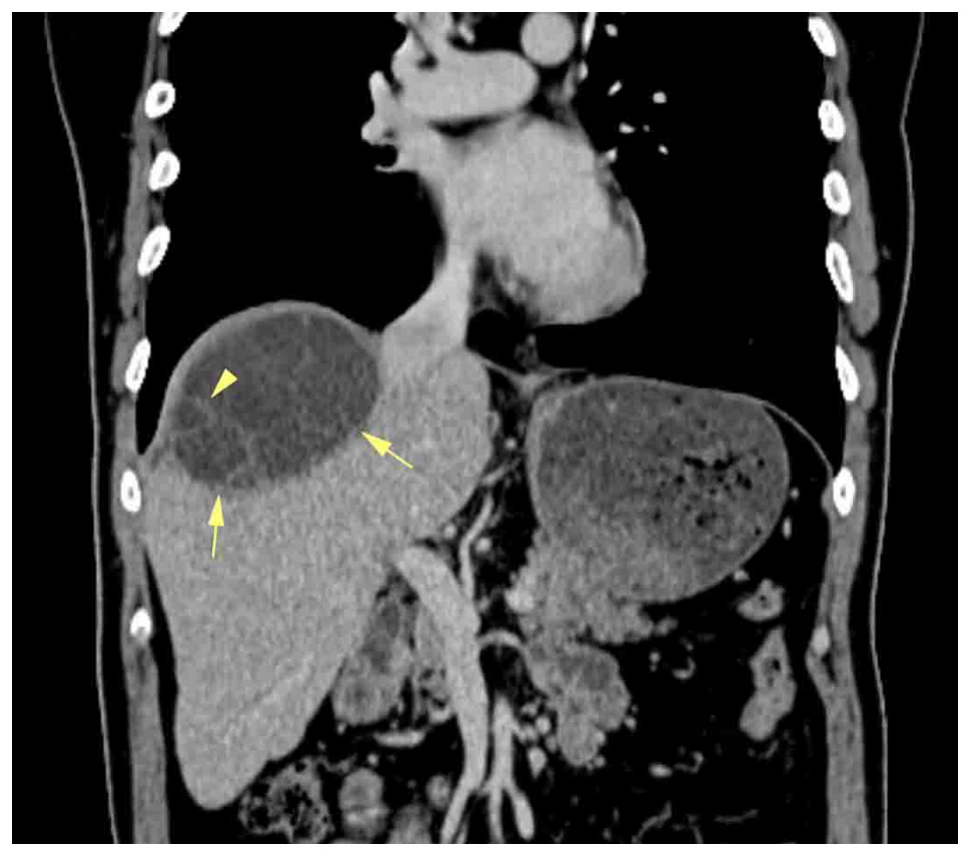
A coronal reformatted computed tomography scan revealed a large, thick-walled subcapsular cystic lesion (arrows) with multiple thin internal septations (arrowhead), located at the liver dome just beneath the right hemidiaphragm.
